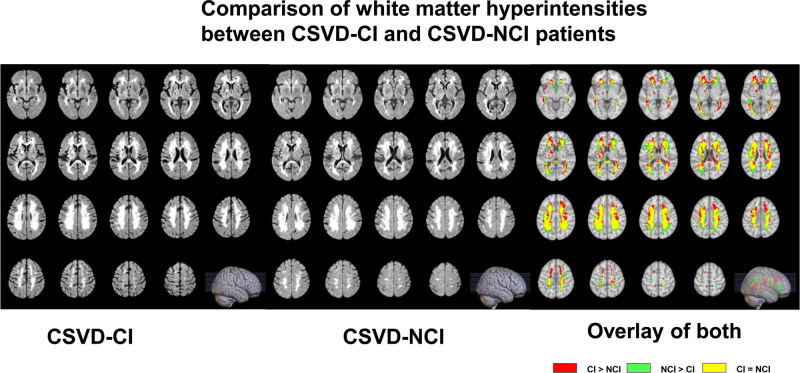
Comparison of white matter hyperintensities between cerebral small vessel disease (CSVD)-CI and CSVD-NCI patients (CSVD-CI indicates patient 1 and CSVD-NCI indicates patient 2). In both cases, the magnetic resonance imaging (MRI) showed a large fusion of cerebral white matter high signals, which were classified as grade 3 according to the Fazekas classification. Comparison of patient 1 and patient 2 showed that there were more overlapping areas of cerebral white matter high signal, and by superimposing MRI, it could be seen that patient 1’s cerebral white matter high signal was higher than that of patient 2, which was concentrated in the bilateral frontal-parietal lobes, the corpus callosum, the parietal ventricles, and the thalamus, while the cerebral white matter high signal of patient 2 was higher than that of patient 1, which was concentrated in the subcortical area. CI = cognitive impairment, NCI = noncognitive impairment.
